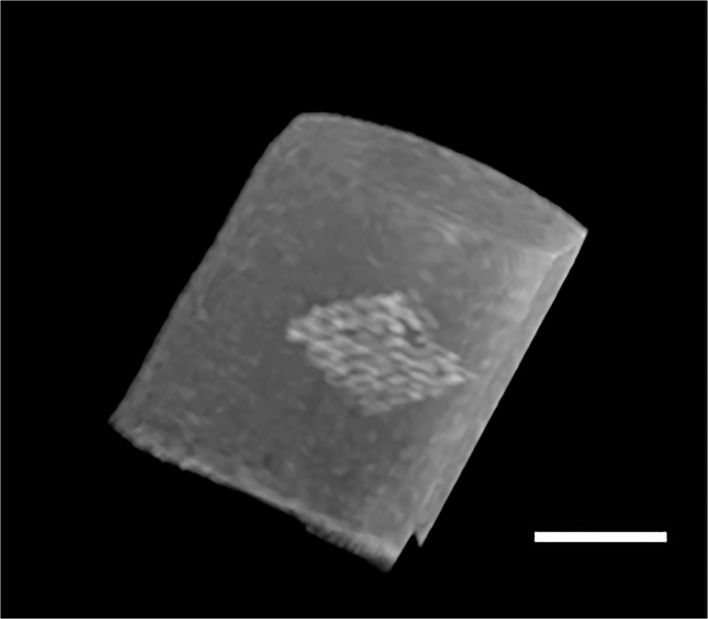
MRI images of the mesh in vitro. Gd-PMA coated mesh with a 3T MRI device, reconstruction was obtained with osiriX software. Scale bar 1 cm.
